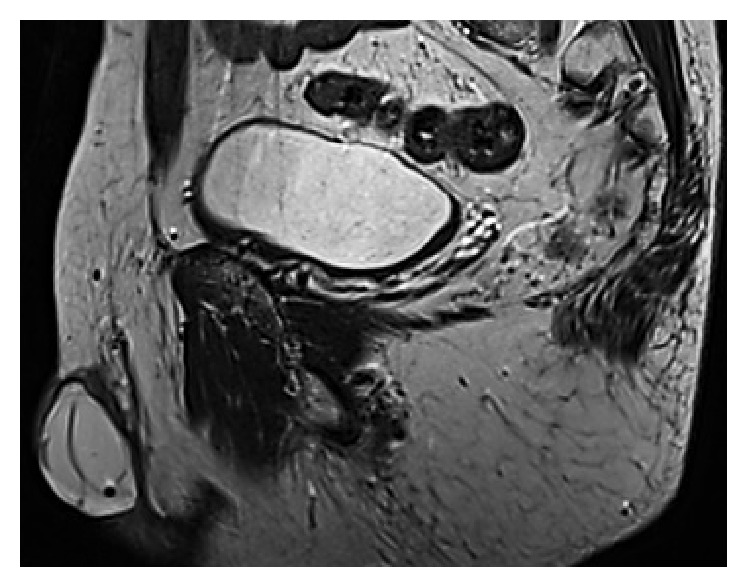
MRI showing prosthesis with linguini sign (free-floating loose thread).
